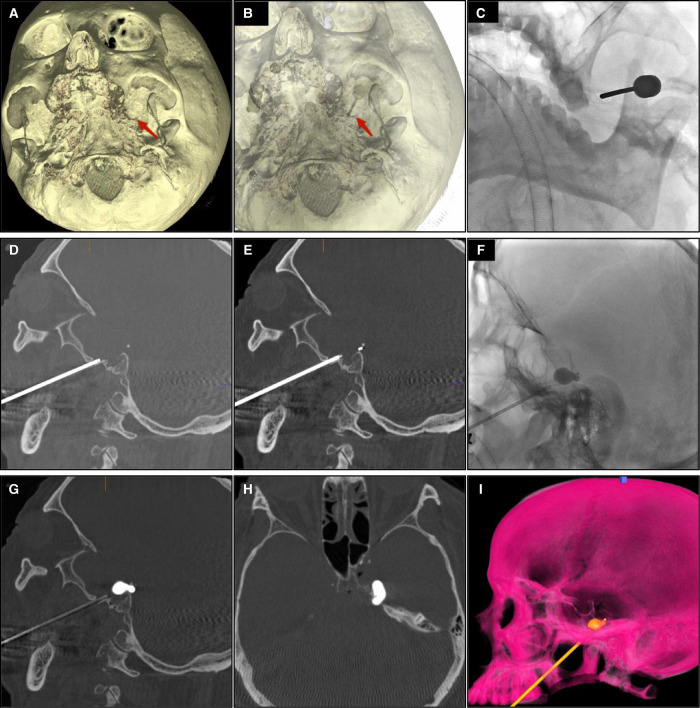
(A) Intraoperative 3D reconstruction of the skull immediately after Dyna CT scan. (B) The FO puncture was performed under the 3D skull image. (C) The DSA dynamic fluoroscopy. (D) Dyna CT scan was performed to adjust the direction and depth of the metallic HI to the correct position. (E) The distal mark of the FC was located at the apex of the Meckel chamber. (F) The balloon was fully inflated and pear-shaped. (G) Balloon morphology under Dyna CT (sagittal view). (H) Balloon morphology under Dyna CT (axial view). (I) Balloon morphology in 3D rendering.
